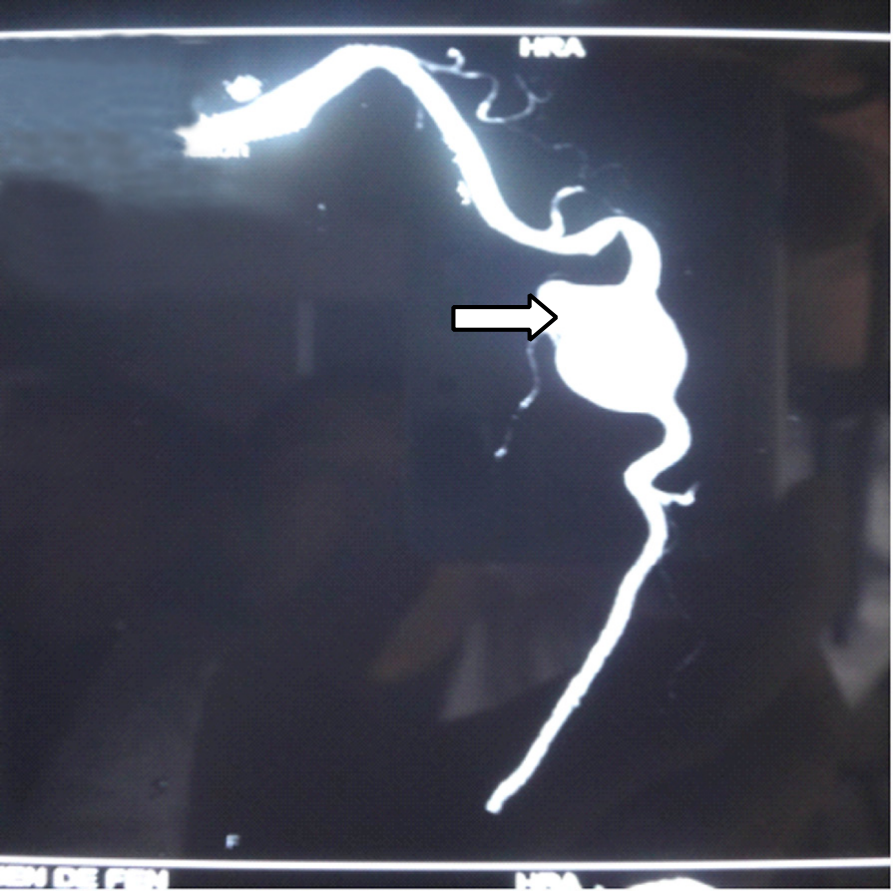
Computed tomography (CT) angiography revealed a 3×5cm size true aneurysm of the left axillary artery (arrowed).
